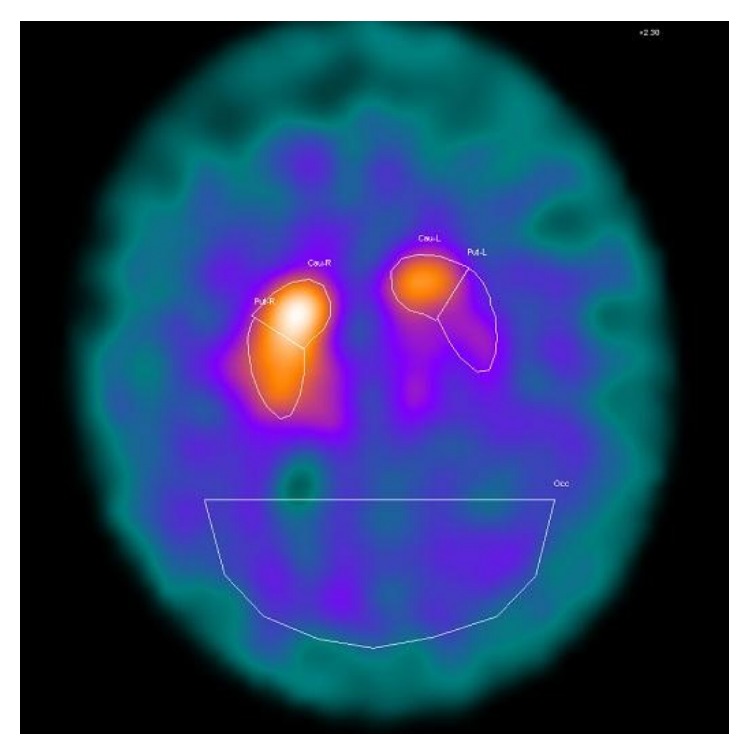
^123^I-FP SPECT showing a reduced tracer binding in the left striatum, with a more marked reduction in the putamen.
